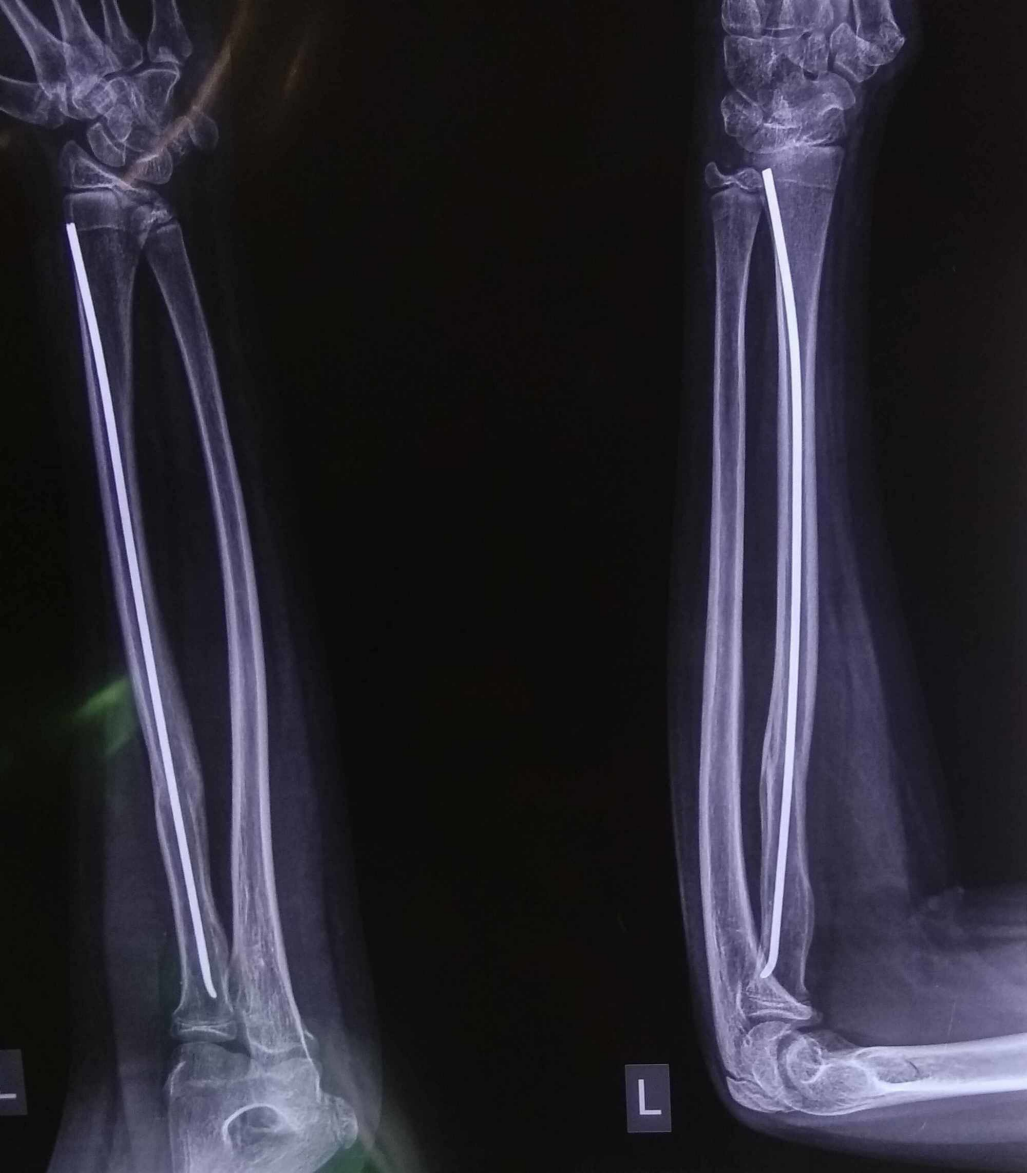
Anteroposterior and lateral radiograph of the united radial fracture with in situ elastic nail.
